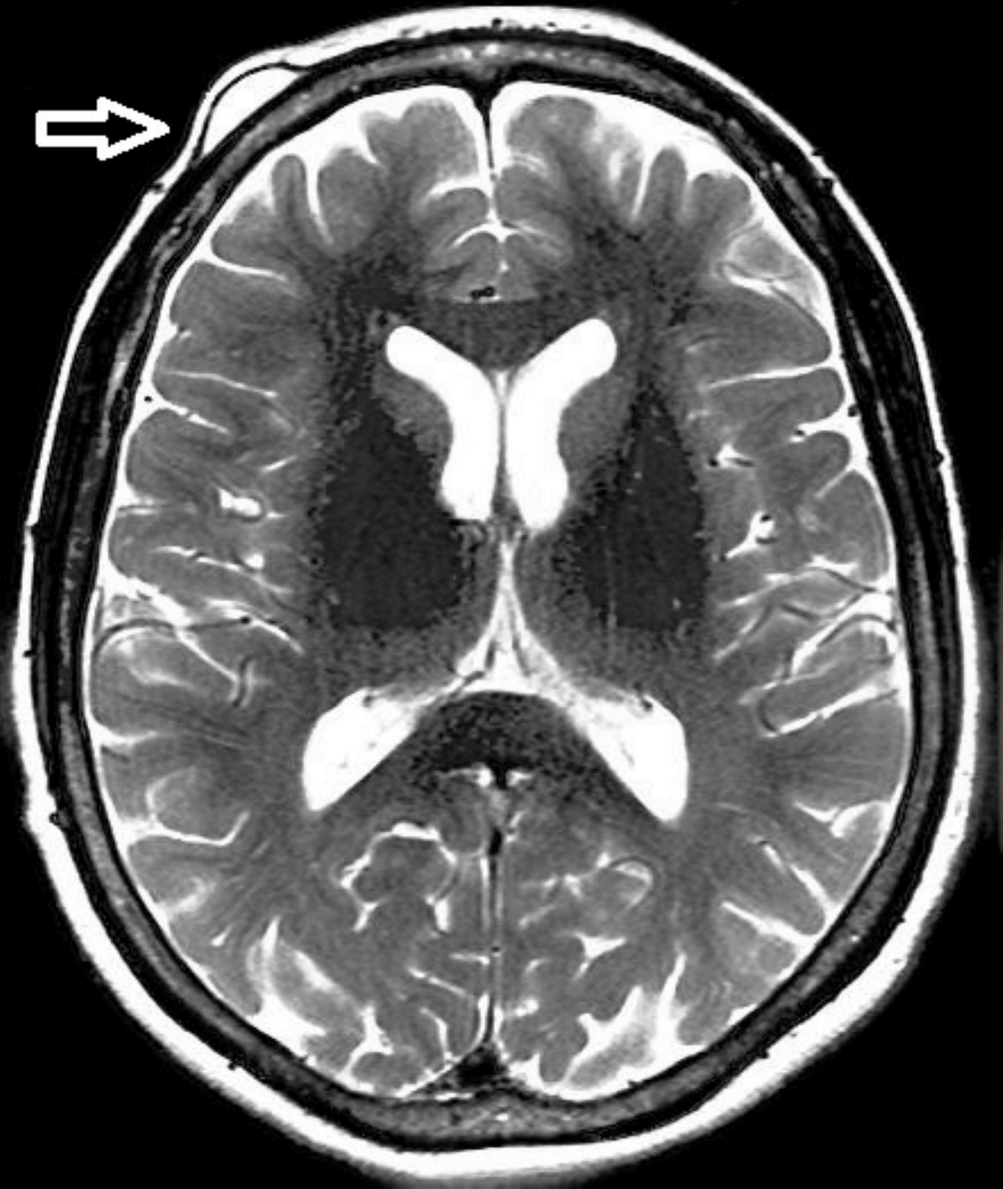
T2-weighted sequence of MRI of the head region showing oval-shaped hyperintensity in the left frontal region (arrow)
